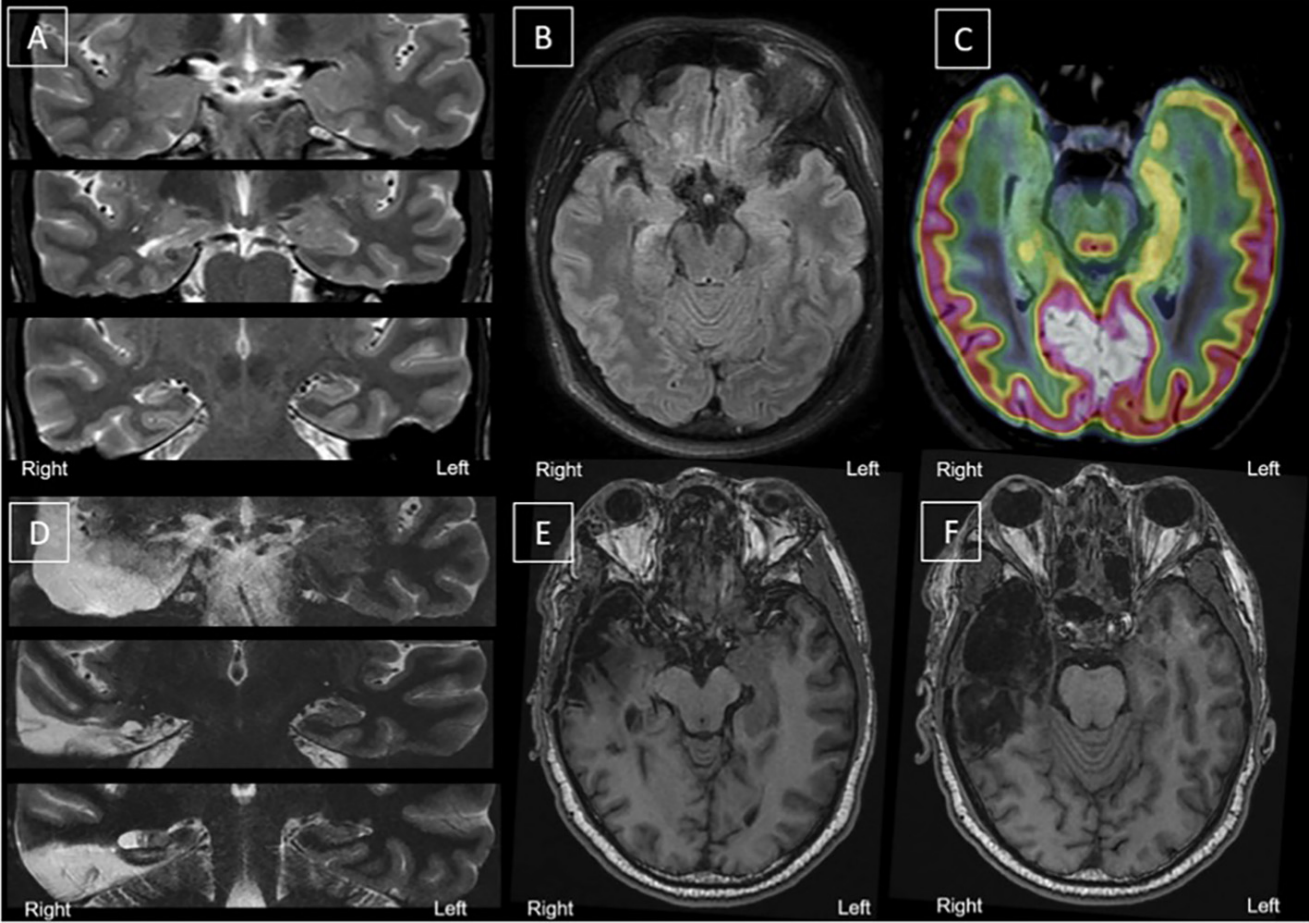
Morphological and metabolic imaging before and after surgery. (A–C) Before surgery. (D–F After surgery. (A): Coronal slices, top to bottom, anterior to posterior hippocampus—T2 MRI; (B) Axial Slices (T2 MRI) showing right hippocampal sclerosis; (C): Fusion between T2 MRI (hippocampal axis) and FDG-PET (hippocampal axis) showing right medial hypometabolism. (D) Coronal slices, top to bottom, anterior to posterior hippocampus—T2 MRI; (E–F) Axial slices (T1 MRI) of the right medial temporal area after selective surgery. Surgery left a porencephalic cavity with gliosis, limited to the right temporal region; right meningeal thickening and T1 contrast enhancement near amygdalo-hippocampal area (not shown). FDG-PET, F-18-fluorodesoxyglucose positron emission tomography; MRI, magnetic resonance imaging.
